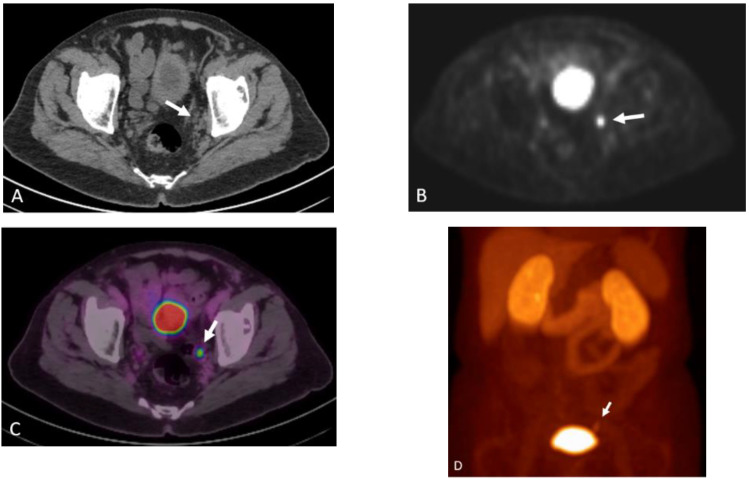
NECT (A) shows a round hypoattenuating lesion along the left pelvic sidewall (arrow). Corresponding 18F DCFPyL PET (B), fused PET/CT (C), and MIP (D) images shows that this lesion demonstrates radiotracer uptake. Upon further review, this was found to be excreted radiotracer in the left distal ureter.
